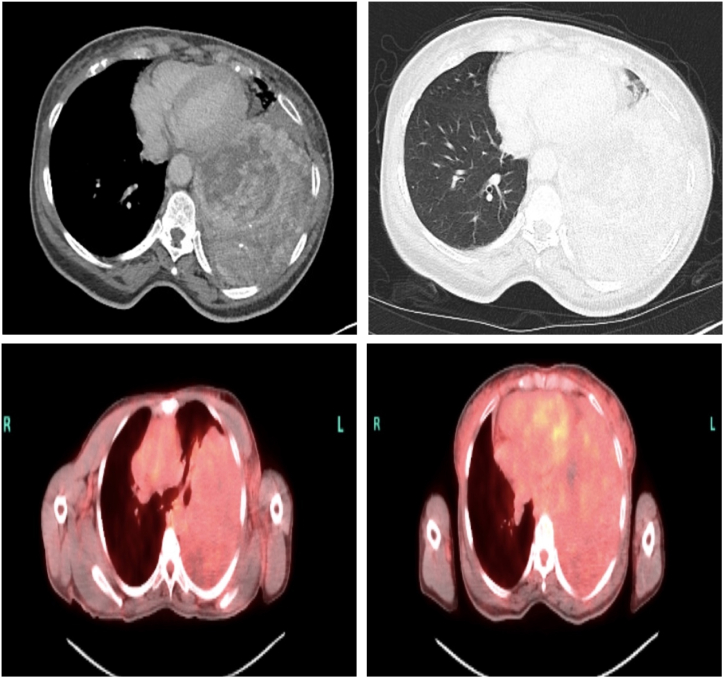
CT of the chest with and without contrast and PET CT of the chest.* A large mass within the left hemithorax, extending from the mid-lung to the lung base and into the costophrenic angle. The mass is diffusely heterogeneous, with linear enhancing solid components and areas of thick-walled, septated cystic-necrotic changes, particularly in the superior posterior aspect. No significant osseous erosion or invasion of the chest wall is identified.
